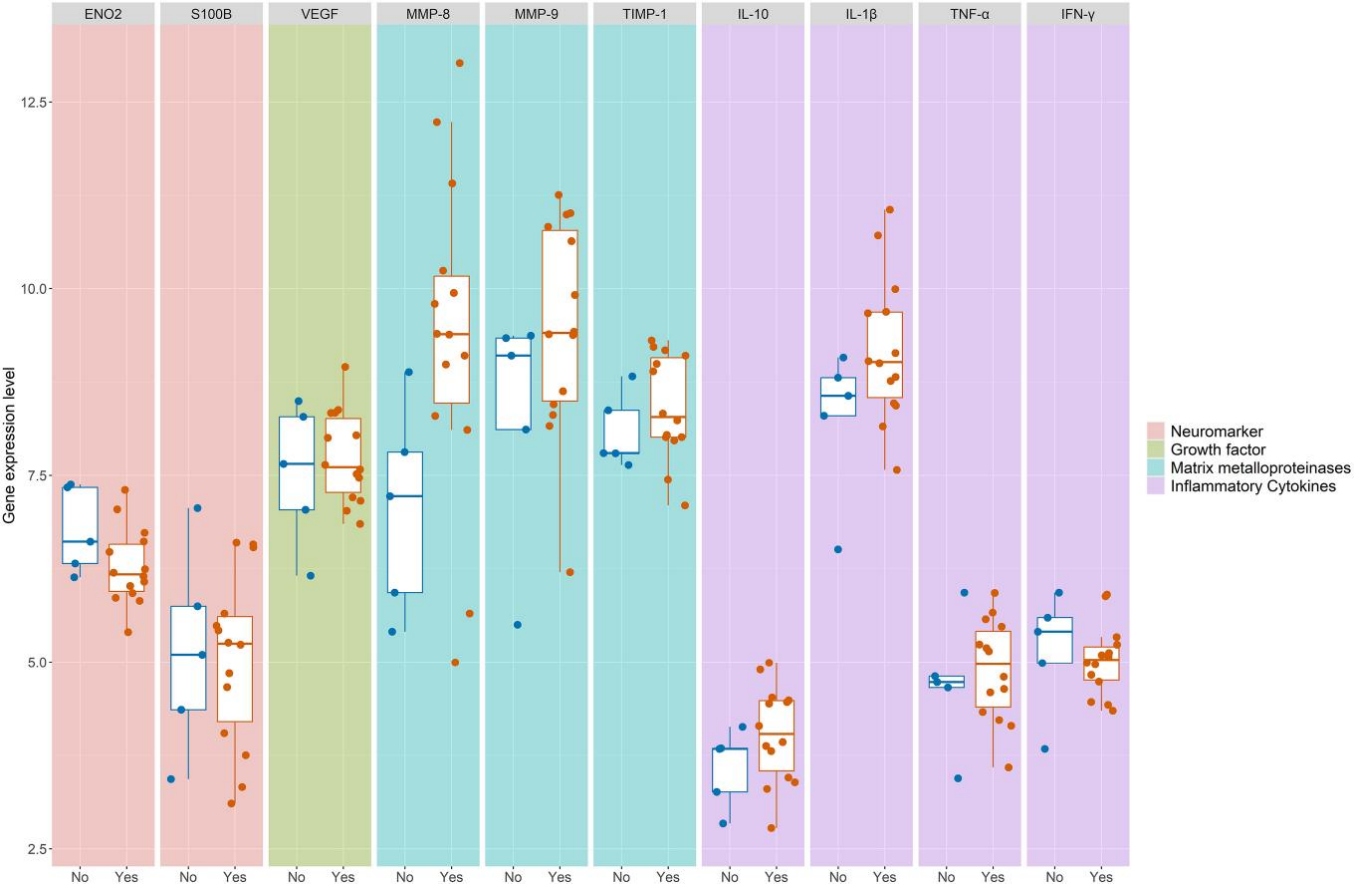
Expression of selected inflammatory mediators in the whole blood of children with infarcts and TBM infection. Nineteen children with definite, probable, or possible TBM had baseline magnetic resonance imaging and whole blood RNA sequencing for neuromarkers (ENO2, S100B), inflammatory cytokines (IL-1β, IFN-γ, TNF-α, IL-10), growth factors (VEGF), and matrix metalloproteinases (MMP-8, MMP-9, TIMP-1). Fourteen children had radiologically determined infarcts and 5 did not. Dots, individual patient data; boxes, IQR; line within box, median; whiskers, values up to 1.5 times IQR. MMP-8 expression was increased in children with cerebral infarcts. Abbreviation: TBM, tuberculous meningitis.
